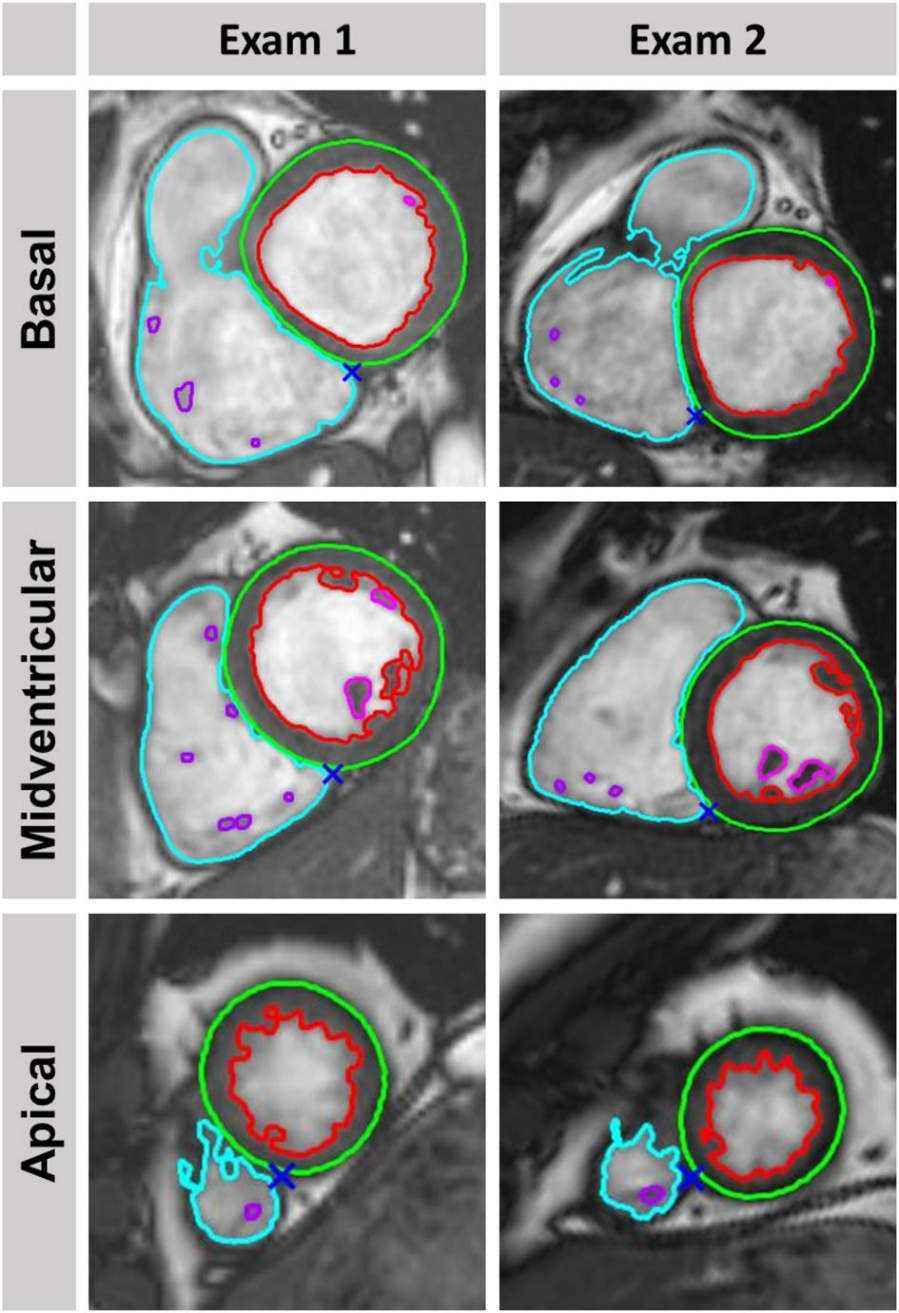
Fully automated biventricular segmentation. The figure depicts automated biventricular volume assessments for a representative volunteer at the basal, midventricular and apical level at baseline MRI (Exam A) and follow-up MRI (Exam B). Higher inter-study variability may potentially be induced by the basal segmentation in the example.
